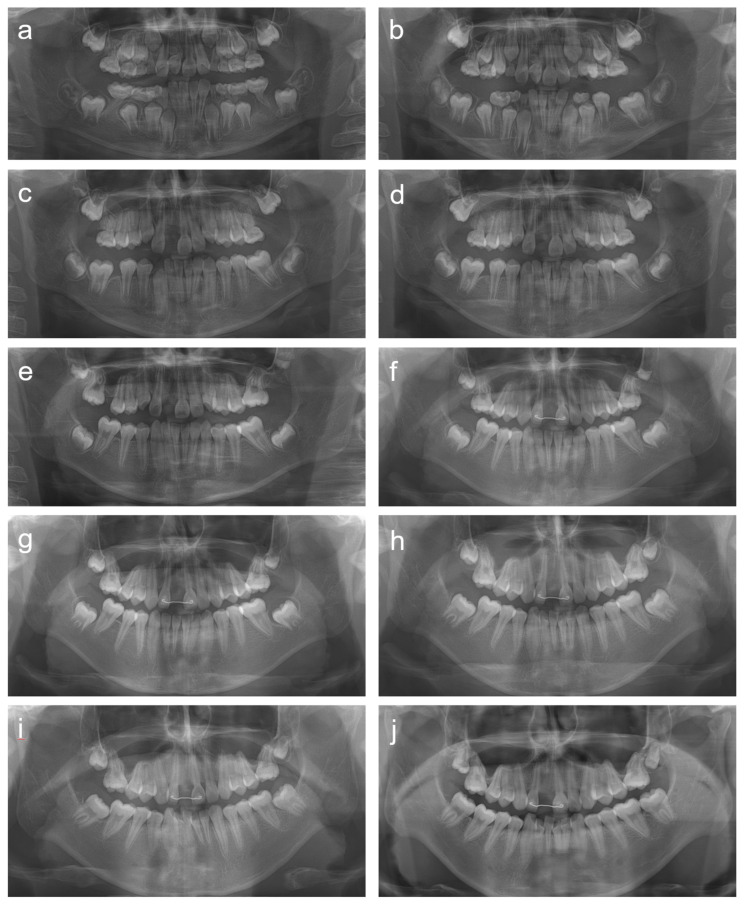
Panoramic radiographs of patient 3. (a) Panoramic radiograph at age 9 years. (b) Panoramic radiograph at age 9 years 9 months. (c) Panoramic radiograph at age 10 years 8 months. (d) Panoramic radiograph at age 11 years 2 months. (e) Panoramic radiograph at age 12 years 3 months. (f) Panoramic radiograph at age 13 years 3 months. (g) Panoramic radiograph at age 14 years 3 months. (h) Panoramic radiograph at age 15 years 3 months. (i) Panoramic radiograph at age 16 years 3 months. (j) Panoramic radiograph at age 17 years 4 months.
